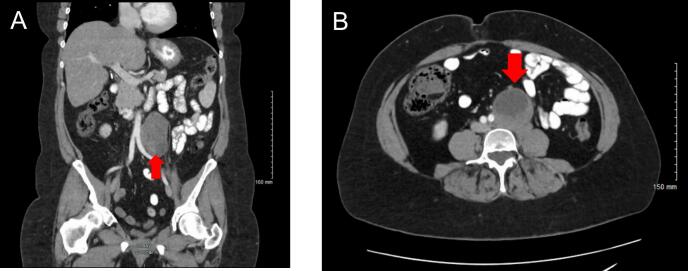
CT views of the abdomen A: Coronal section of the abdomen showing soft tissue density B: Transverse section of the abdomen showing soft tissue density.
